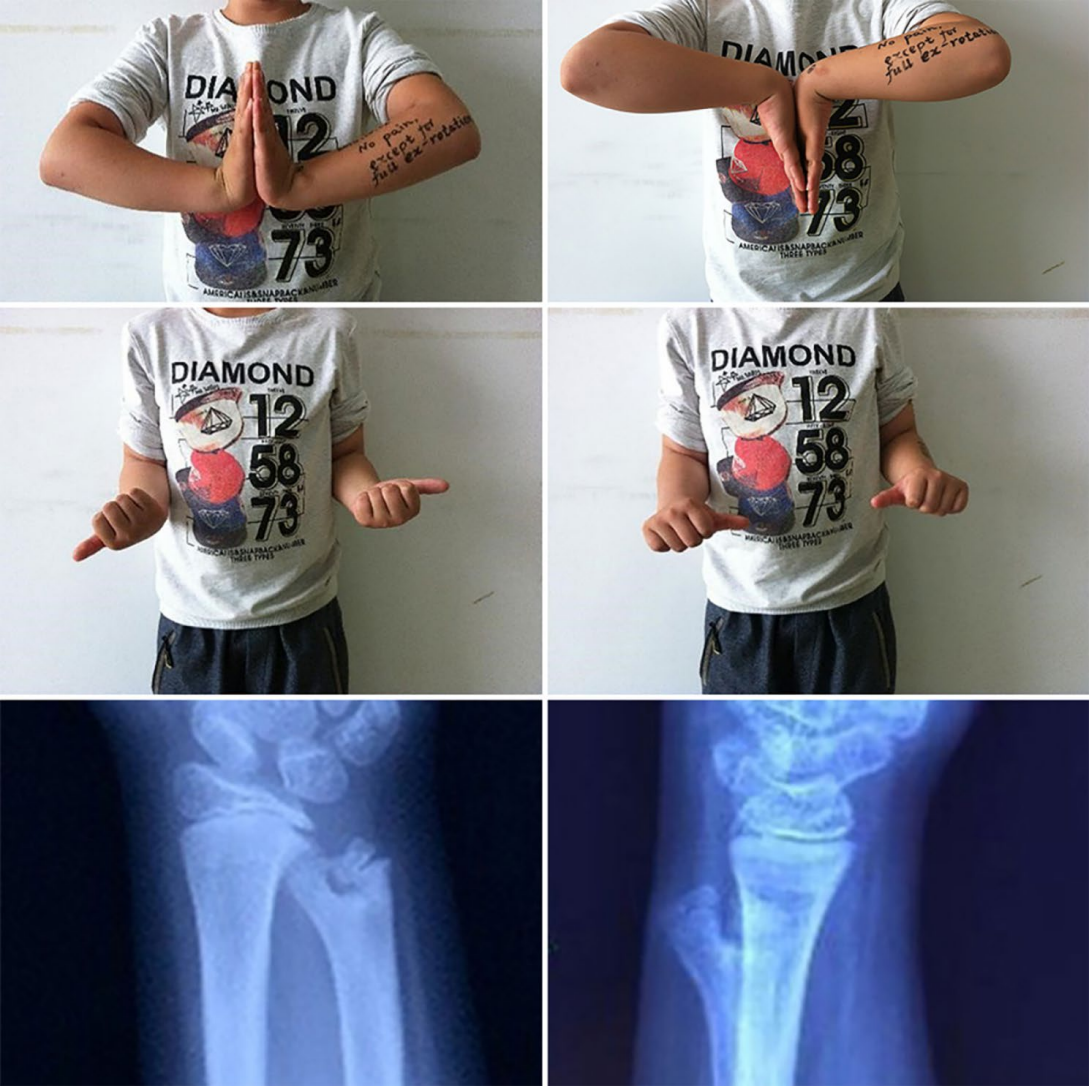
Functional activity and X-ray of the patient’s hand at 7 months post-injury follow-up
